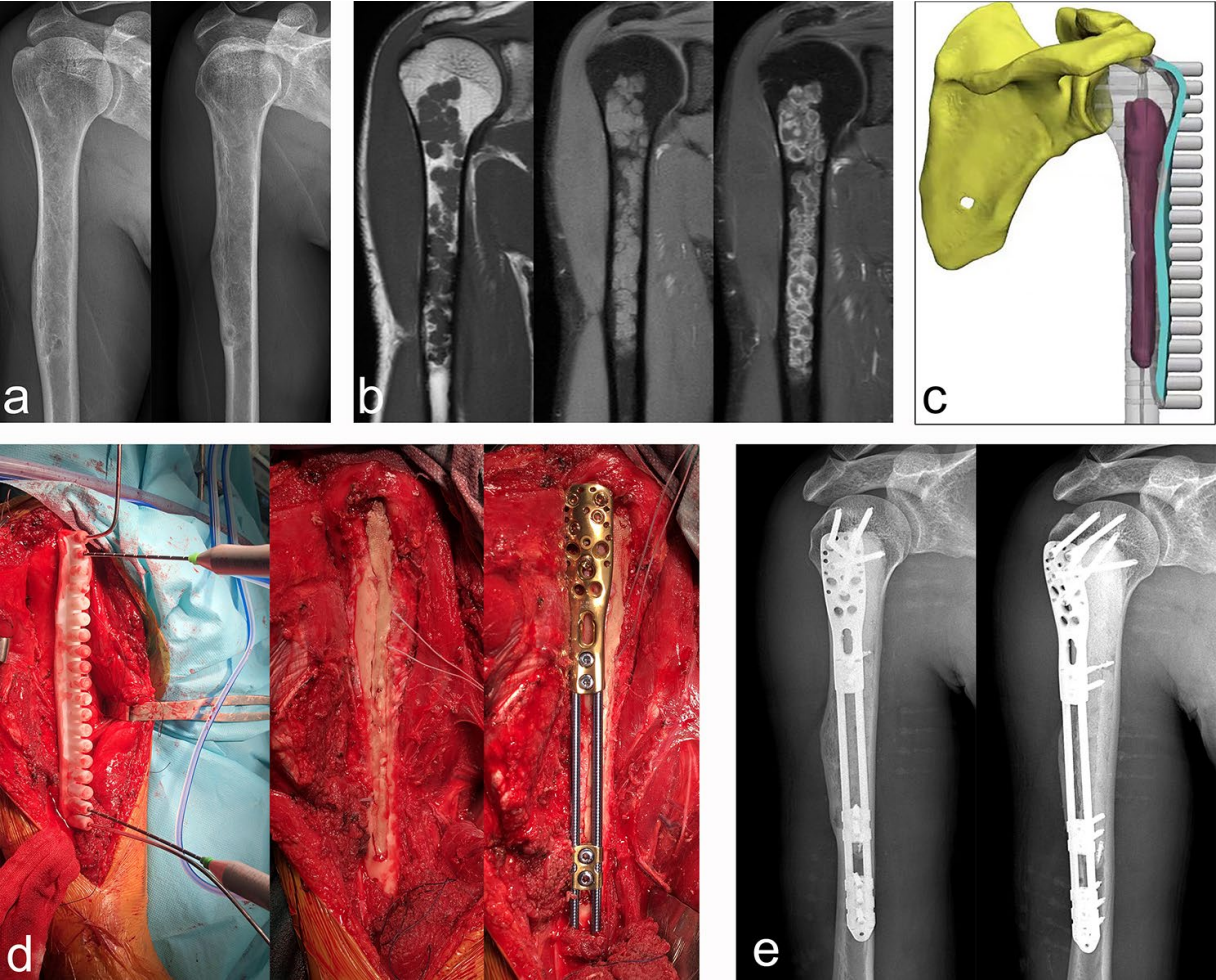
Typical cases of intramedullary chondrosarcoma of proximal humerus. (a) Anteroposterior and lateral X-rays showed distension of the proximal humerus without intramedullary calcification. (b) MRI showed typical chondrogenic tumor signal changes with inhomogeneous enhancement. (c) Computer aided design of microwave ablation guide plate. (d) The following procedures were two-needle microwave ablation assisted by 3D printing guide plate, cement filling after extended curettage, and prophylactic internal fixation with anatomic bridging internal fixation system for proximal humerus. (e) Postoperative anteroposterior and lateral X-ray
